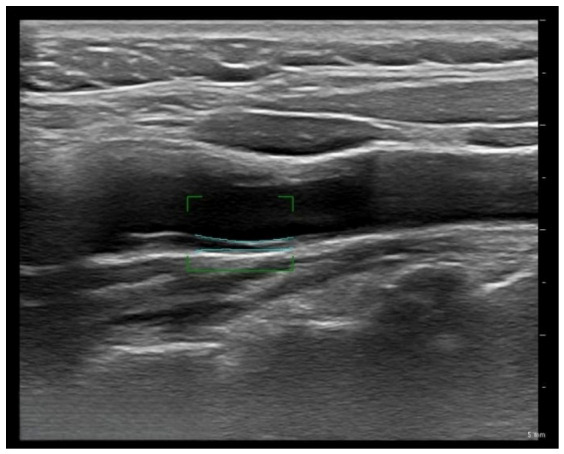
Example of a carotid ultrasound showing an IMT value at the upper limits of normal (0.96 mm), in a patient with CV risk factors, supporting the role of vascular ultrasound in the assessment of subclinical vascular damage. The green box and the blue line indicate the site where the IMT measurement was performed.
